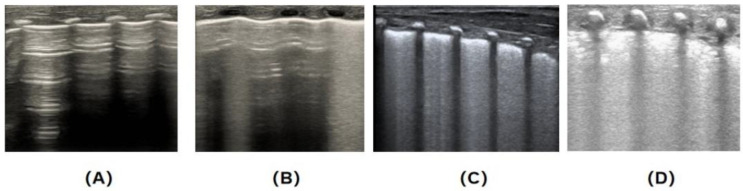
Calculation of Lung Ultrasound Score [15]. Segmentation: Each lung is divided into three regions—anterior (L12/R12), lateral (L34/R34), and posterior (L56/R56)—and both lungs comprise a total of six regions. The total score ranges from 0 to 18. A higher score indicates more severe lung involvement. The detailed scoring criteria are as follows: (A) 0 points (normal aeration): Characterized by A-lines with sporadic B-lines; (B) 1 point (moderate aeration loss): Including fused B-lines and interstitial syndrome (B-line occupying < 50% of the examined lung field); (C) 2 points (severe aeration loss): With a small amount of localized subpleural consolidation (B-line occupying ≥ 50% of the examined lung field; B-line covers more than half of the intercostal space); (D) 3 points (extensive consolidation): Extensive lung consolidation.
